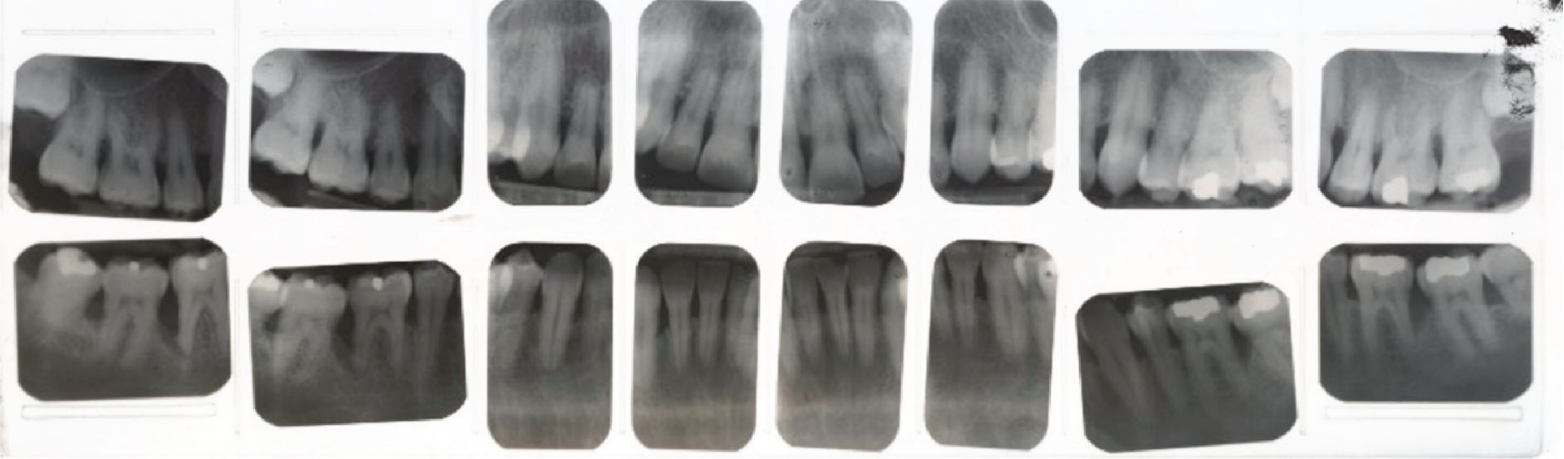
Copy of the original full-mouth radiographic survey (FMRS) taken at the periodontal office, January 9, 1991.
